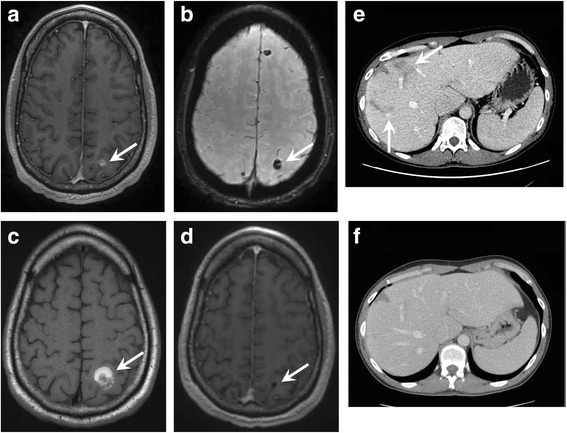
Axial post-contrast FSPGR obtained 1 week after the start of ipi/nivo due to new headaches shows a new 6 mm enhancing (a, arrow) and hemorrhagic left parietal lesion (b, arrow), one of 9 new lesions. 6 week follow-up MRI during ipi/nivo therapy revealed interval hemorrhage of the left parietal lesion (c, arrow) and decreased size of the additional previously enhancing lesions. Axial images from concurrent abdominal CT examinations demonstrate systemic disease response with decreased size and number of hepatic lesions after 6 weeks of ipi/nivo therapy (arrows in e compared to f). After 6 months of therapy, MRI confirmed contraction of the hemorrhagic cavity of the left parietal metastasis (d, arrow) and no new/residual enhancing lesions
